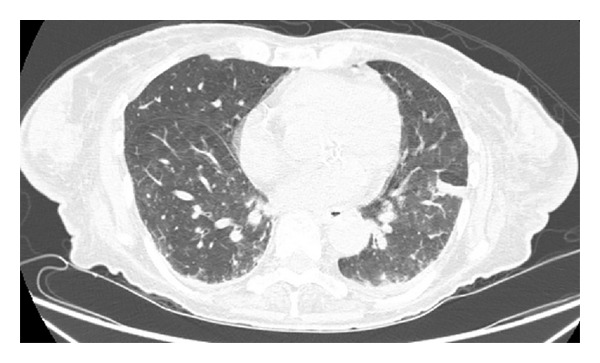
Computed tomography (CT) scan of the chest showed diffuse interstitial thickening and ground glass opacities.
